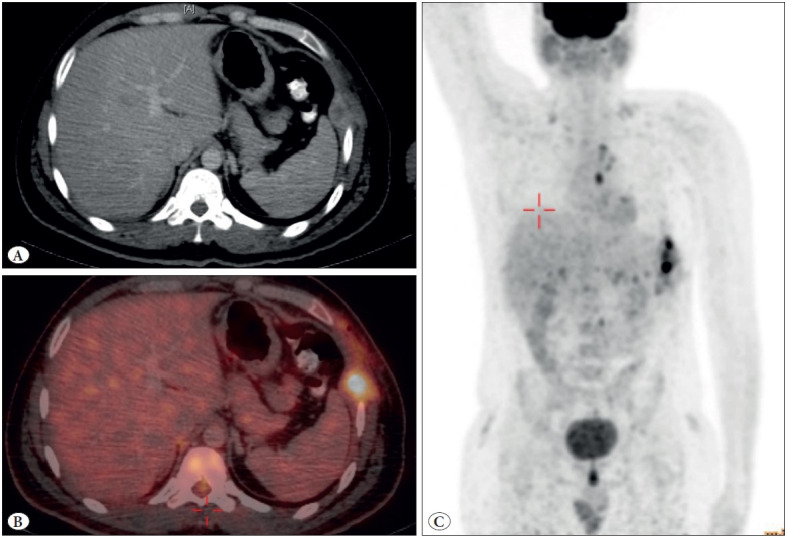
Fluorine-18 fluorodeoxyglucose Positron emission tomography/ Contrast enhanced computed tomography scan images showing an A) ill defined lobulated hypodense soft tissue lesion with peripheral enhancement in the left chest wall at the post-operative site, B) which is metabolically active. The lesion extends along the 7th and 8th intercostal spaces. C) Few metabolically active mediastinal nodules are also noted.
